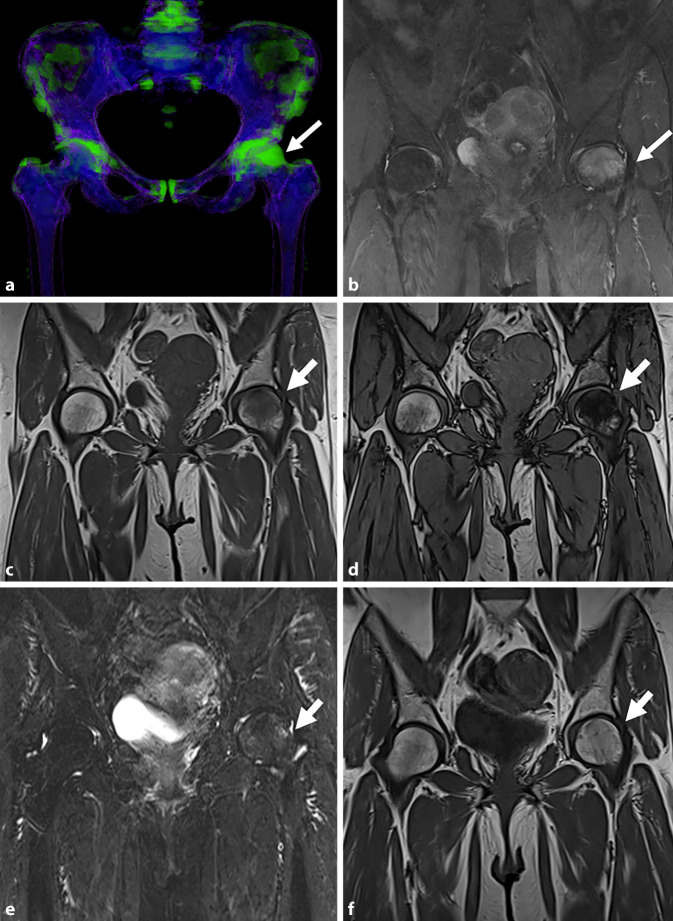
A 55-year-old patient with left hip pain related to reversible left hip bone marrow edema (BME) syndrome. a Frontal view dual-energy computed tomography with bone marrow algorithm shows bone marrow edema of the left femoral head (colored green, *white arrow*). b Fluid-sensitive magnetic resonance sequence performed 2 weeks later confirmed the bone marrow edema (*white arrow*). c T1-weighted Dixon in-phase image shows low signal, isointense to the adjacent muscles (*white arrow*). d The out of phase image shows a drop of signal of > 20%, indicating that there is no marrow replacement and that this is a benign lesion (*white arrow*). e Coronal fluid sensitive magnetic resonance image performed after 5 weeks demonstrates near complete resolution of the BME (*white arrow*). f Coronal T1WI after 5 weeks: the shape of the articular surface is maintained (*white arrow*)
